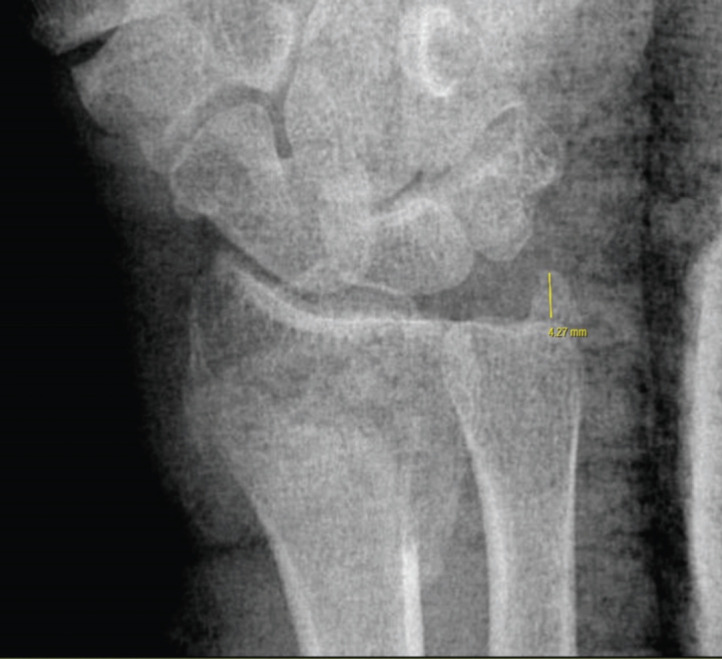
Measurement of an ulnar styloid fracture fragment on pre-operative radiograph.
